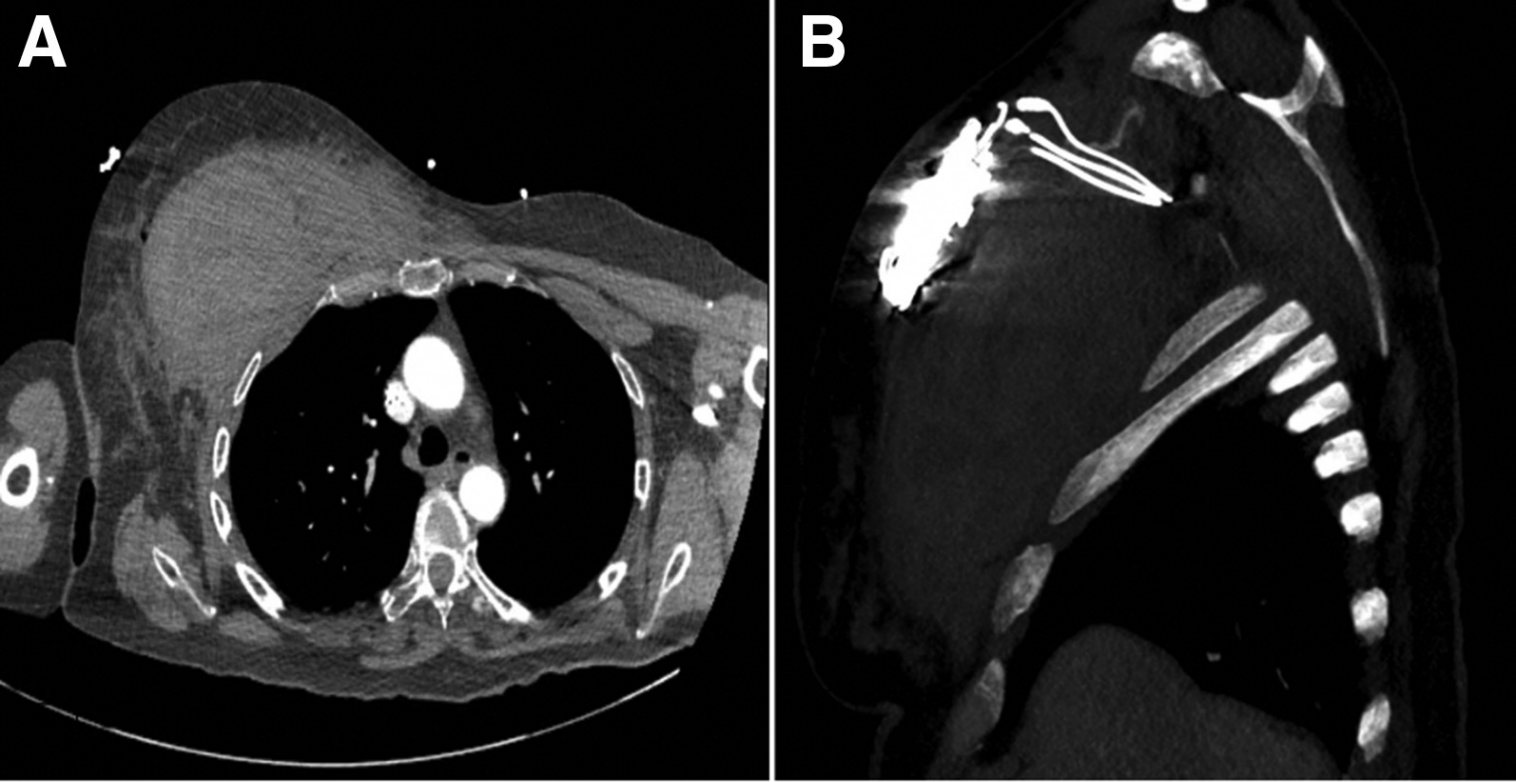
Computed tomography of the chest revealing large right subpectoral hematoma. A: Transverse view. B: Sagittal view.
